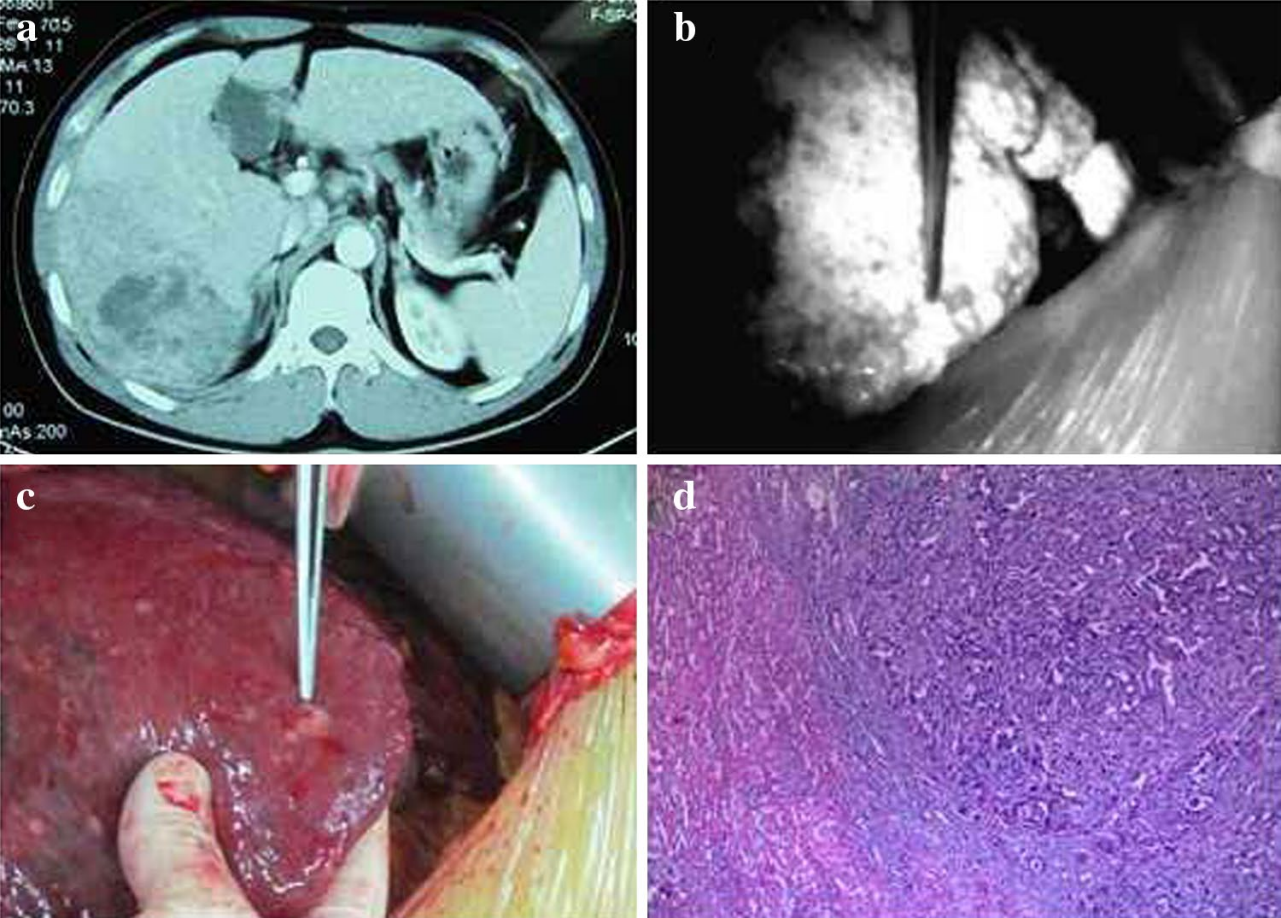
Application of a photodynamic eye for identifying tiny tumors with intraoperative *indocyanine green* administration. Preoperative computed tomography showing a visible VII segment of a liver tumor, and the other segments had no obvious small tumor foci (a), intraoperative photodynamic eye examination of the liver; in addition to known tumors, a 4-mm diameter shadow nodule was visible (b), tumor nodules were found on the liver surface and sent for pathological analysis (c), pathological results confirming hepatocellular carcinoma (d)
